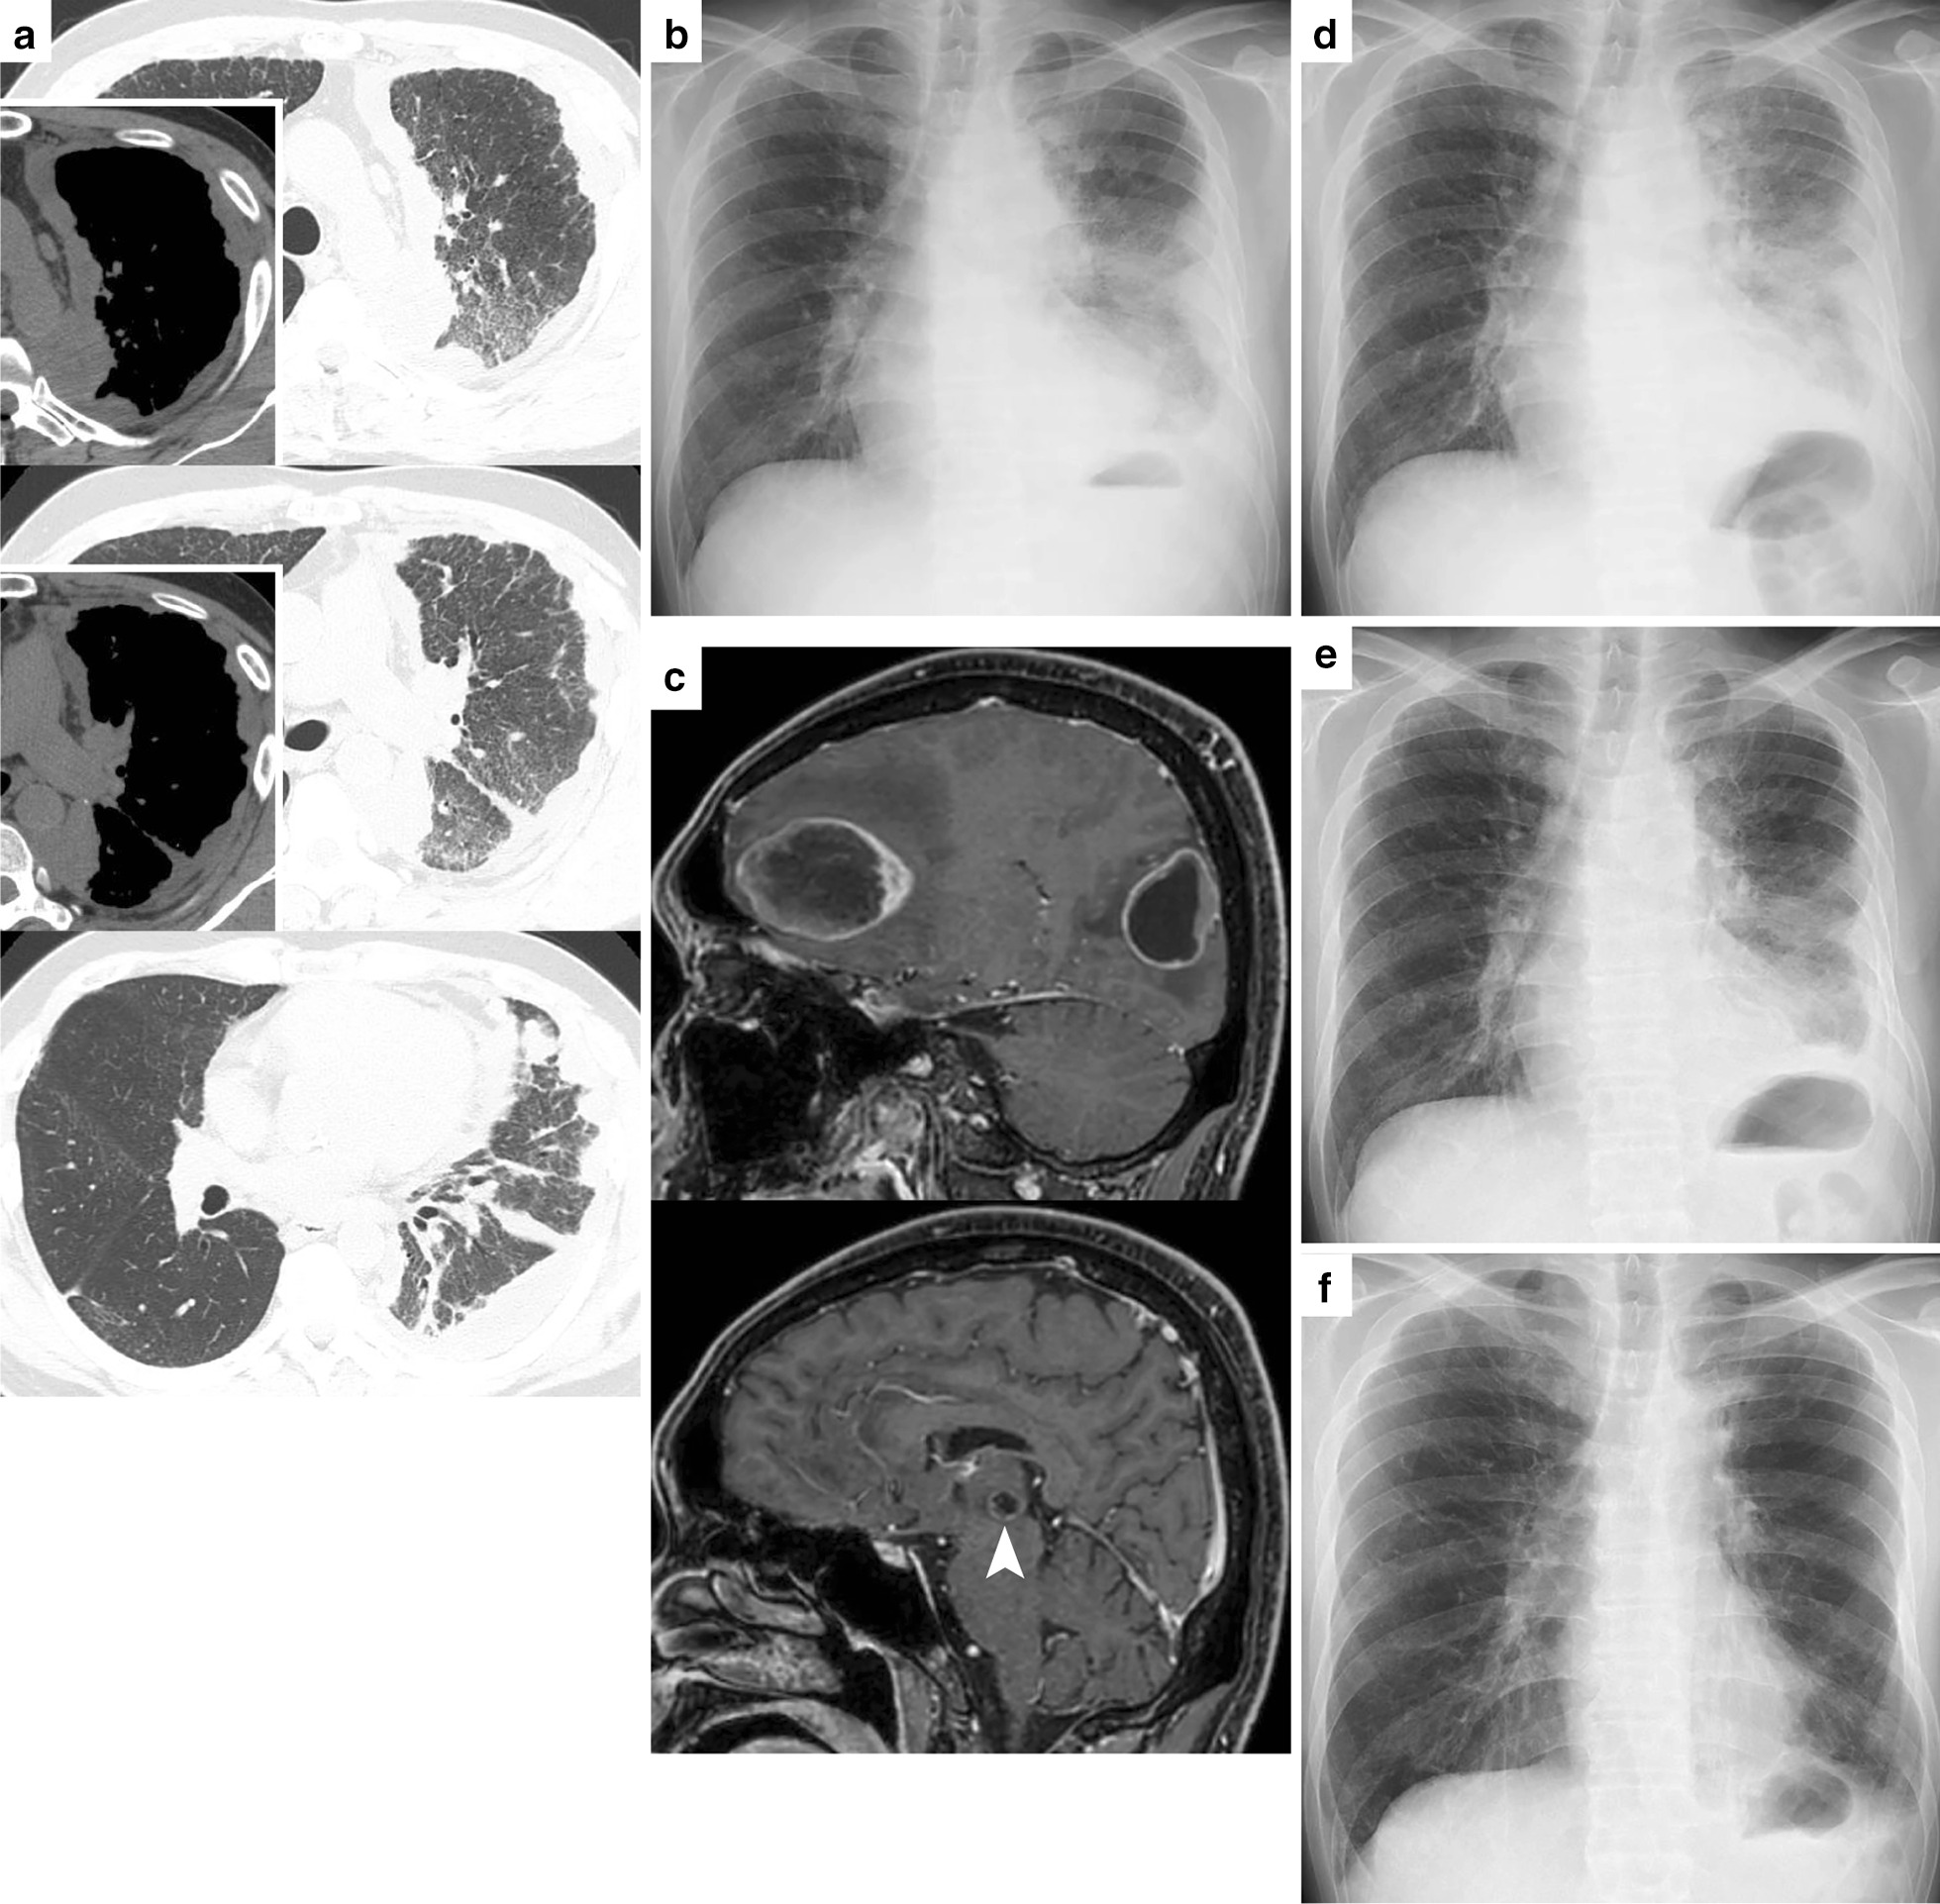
a Chest computed tomography in the planes identical to those in Fig. 1 taken 38 days after the video-assisted thoracoscopy; b chest X-ray taken on the day before the first pembrolizumab administration; c enhanced brain magnetic resonance imaging of the sagittal plane shows metastatic brain tumors. The arrowhead in the lower panel indicates the lesion in the right thalamus; d chest X-ray taken on day 30 of pembrolizumab administration; e chest X-ray taken on day 39 of pembrolizumab administration; f chest X-ray taken after 30 cycles of pembrolizumab
